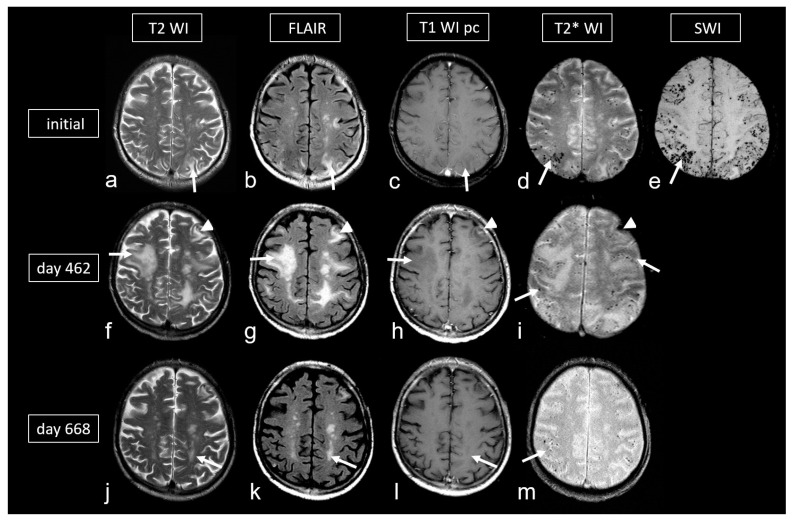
Cerebral amyloid angiopathy-related inflammation (CAA- ri) in a 72-year-old man suffering from subacute deterioration of consciousness and dizziness. (a–e): Initial MRI (upper row; MRI 3.0 T Siemens Magnetom) showing several hyperintense lesions preferentially in the subcortical occipital region ((a,b): arrow) without contrast enhancement on post-contrast T1-weighted images (T1 WI pc; (c), arrow), focal-accentuated microbleeds (MB) on T2* WI ((d), arrow) and susceptibility-weighted imaging (SWI; (e), arrow); note the higher sensitivity for MB on SWI (e) compared to T2* WI (d). (f–i): MRI (1.5 T Intera, Philips Healthcare) at readmission due to subacute severe psychosyndrome after tapered corticosteroid therapy. Multiple occasionally space-occupying hyperintense white matter lesions ((f,g): arrow) without contrast enhancement ((h), arrow) and progressive bilateral MBs ((i), arrow). Note the additional subacute small left frontal intracerebral hemorrhage (ICH; arrowhead). (j–m): Follow-up MRI (1.5 T Intera, Philips Healthcare) after several bouts of intravenous high-dosage methylprednisolone showing distinct regression of white matter lesions ((j,k): arrow) without contrast enhancement ((l), arrow), no significant new hemorrhagic lesions (m).
